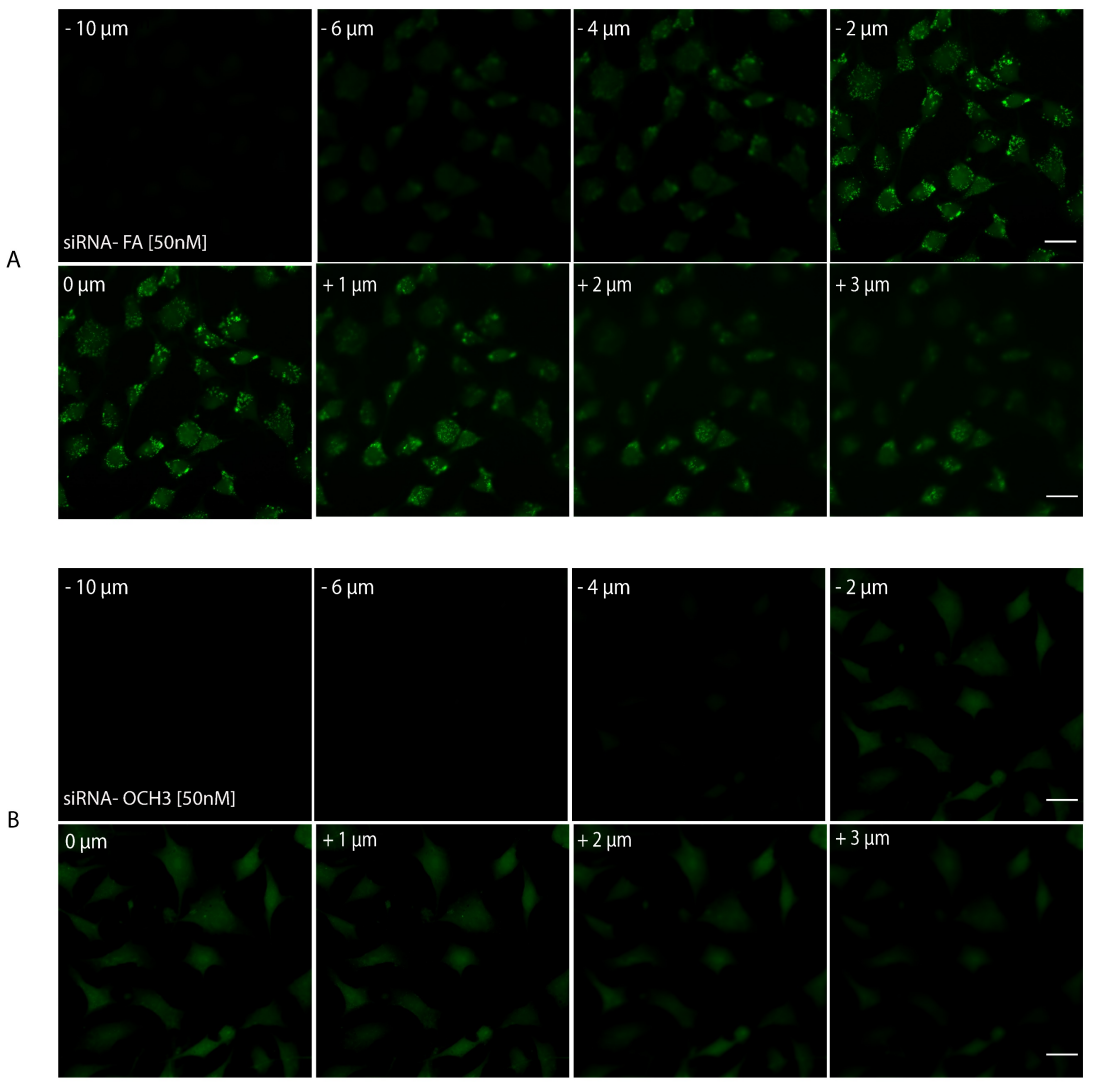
Z-stack imaging of PSMA cells treated with AuNPs-PEG.siRNA (50 nM) vs. AuNPs-FA.siRNA (50 nM). Eight out of 20 sections were chosen as representative sections at a set interval between -10 µm to +10 µm with positive and negative distances relative to the initial focal point of the image. All lasers had the same pinhole. The gain was set at 650 nm for FITC and 550 nm for Hoechst. There is a noticeable decrease in the FITC intensity of AuNPs-PEG.siRNA when compared to AuNPs-FA.siRNA. Furthermore, particles were only visible in sections ranging from -2 µm to + 1 µm in cells incubated with AuNPs-PEG-FA.siRNA (A); particles were absent in cells incubated with AuNPs-PEG.siRNA. The scale bar was set at 20 µm (n = 3).
